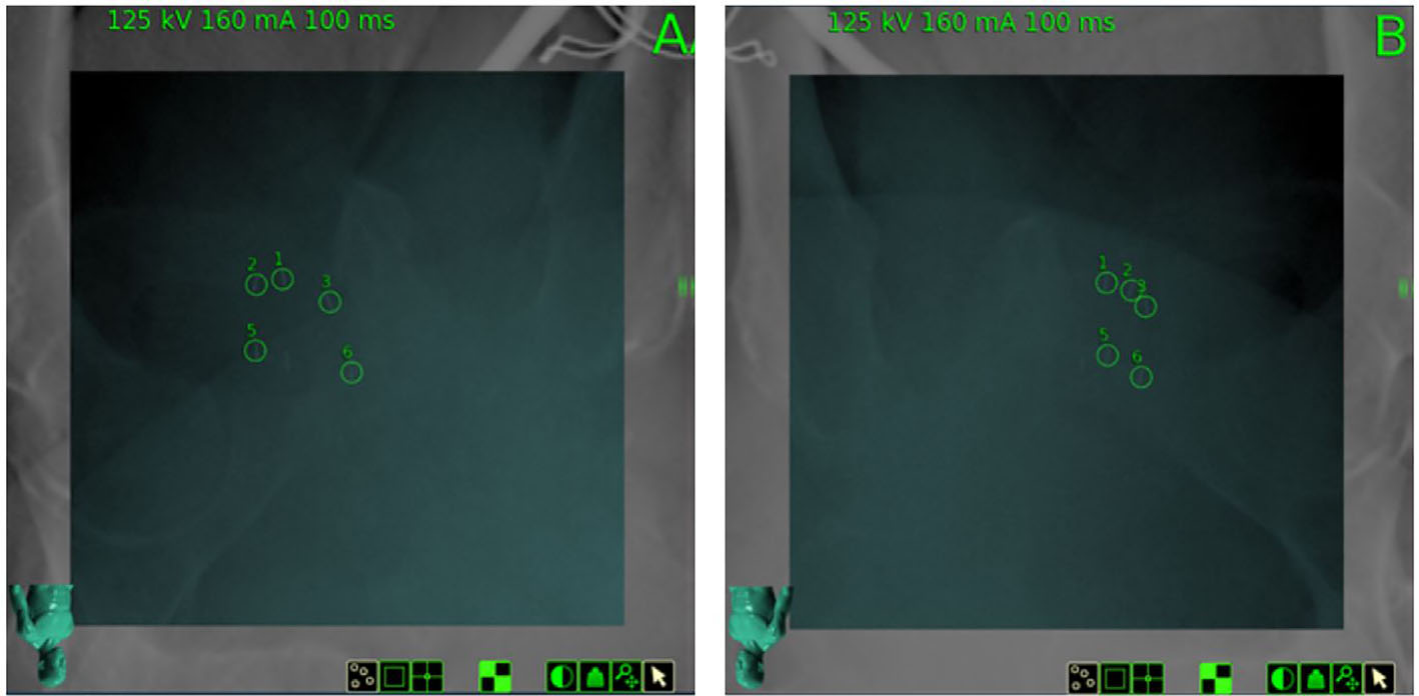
Digitally Reconstructed Radiographs (DRR) on day of treatment from planning CT and paired orthogonal X-Rays (Camera A and Camera B) obtained on the day of treatment, demonstrating gold fiducial alignment for 6D tracking. SpaceOAR Vue™ is not visible.
